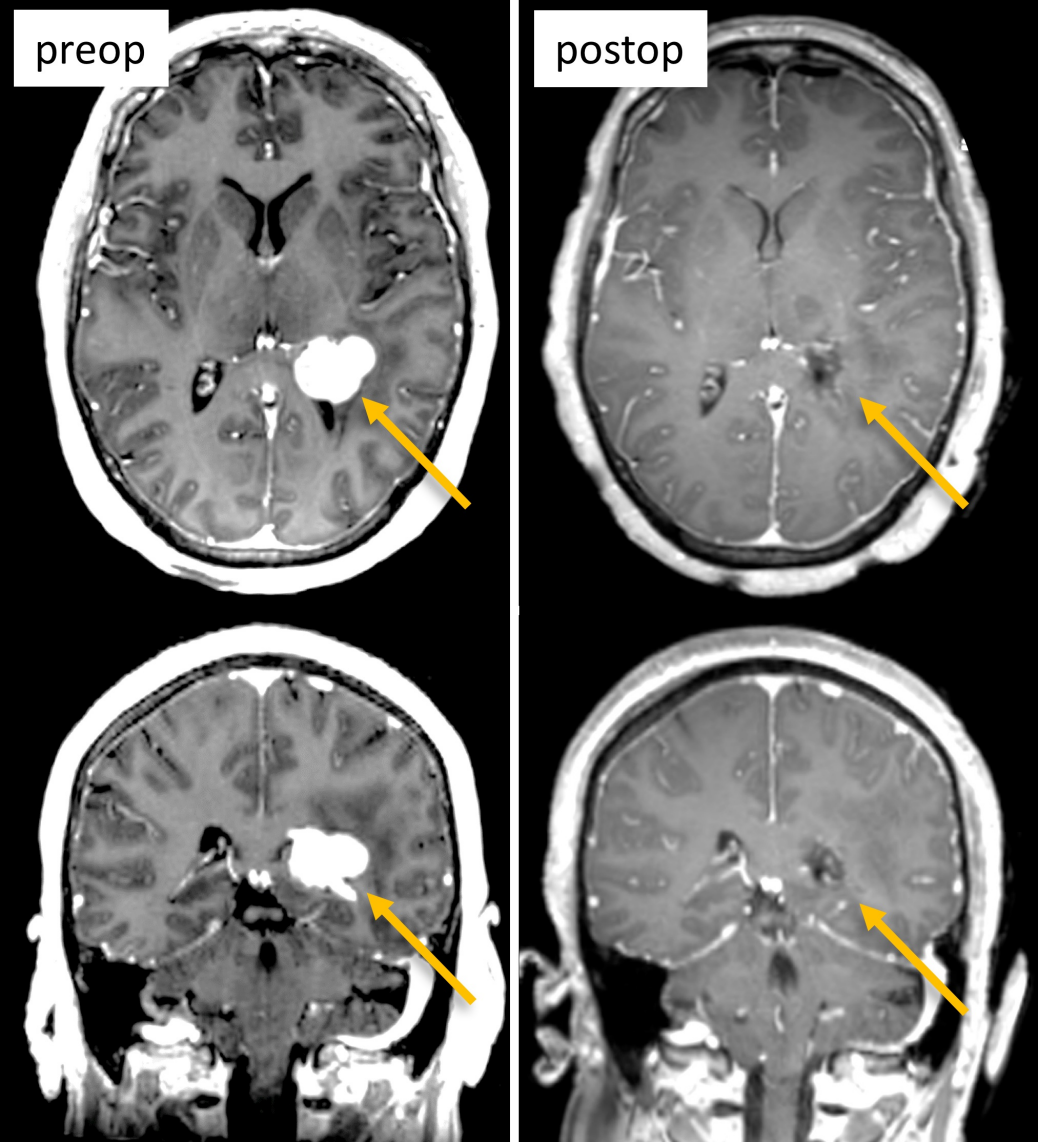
Pre- and postoperative MRI (T1 with contrast, axial top row, coronal bottom row) showing the lesion in the apex of left lateral ventricle (left column, arrows) and gross total resection (right column, arrows). MRI: magnetic resonance imaging
